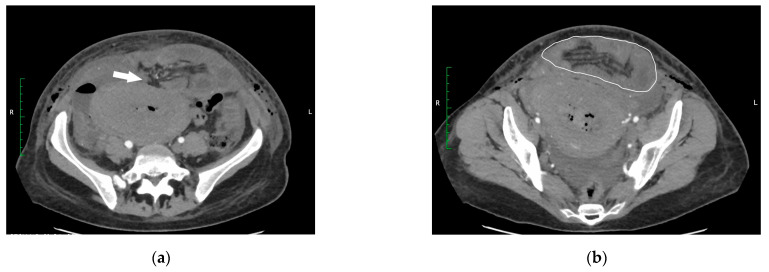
(a) CT scan showing an infraumbilical abdominal wall defect (white arrow). (b) Eventration of the small bowel (white outline).
